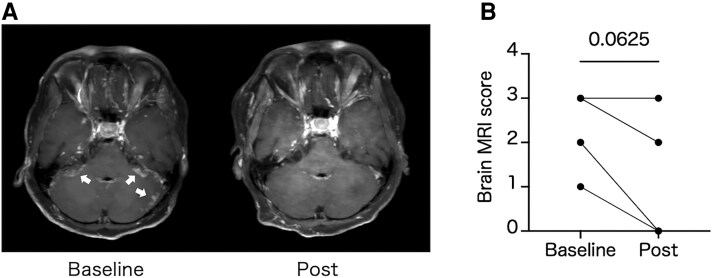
Improvements in MRI inflammatory findings are noted at 1 m following corticosteroid therapy. *A*, Representative post-contrast FLAIR image at the time of PIIRS diagnosis and 1 m after corticosteroid therapy. White arrows indicate cerebellar leptomeningeal enhancement. *B*, Brain MRI score (n = 6). Abbreviations: MRI, magnetic resonance imaging; PIIRS, post-infectious inflammatory response syndrome.
